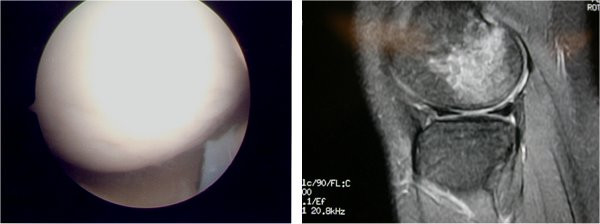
Right: MRI suggesting damage at the articular cartilage. Left: At arthroscopy, the cartilage appeared normal.
